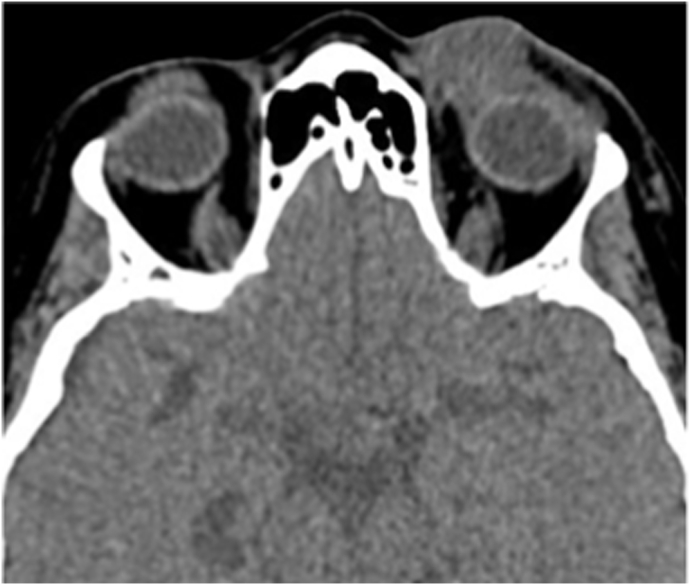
Initial presentation of an elderly Caucasian female with EBV-positive DLBCL. CT scan showing a left orbital lesion corresponding to the left upper lid lesion.
